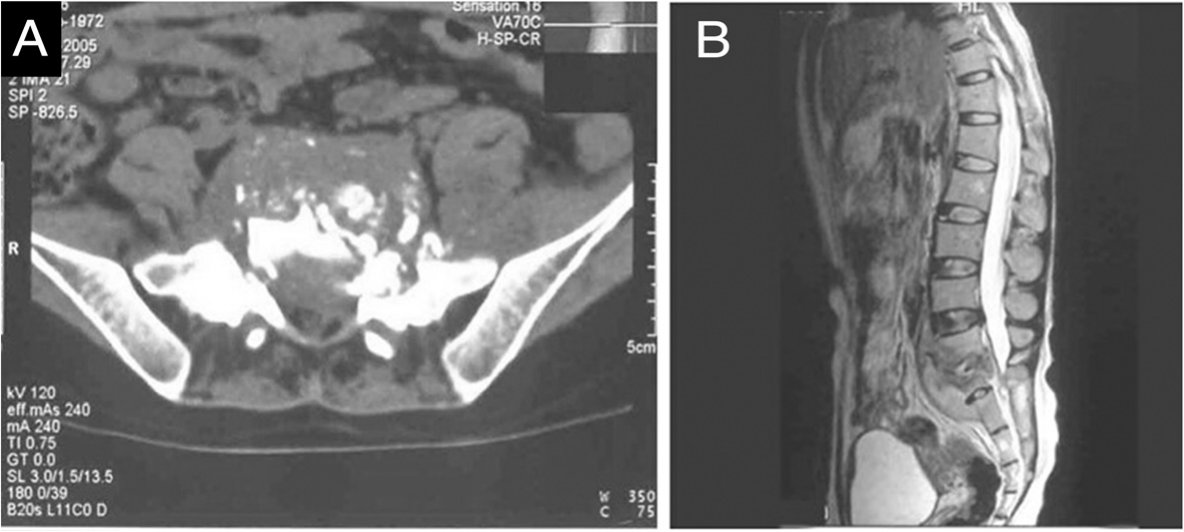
CT and MR images showing bone union of grade I. (A) CT image showing S1 bone destruction, presacral cold abscess, and sequestrum. (B) MRI showing L5–S1 vertebral signal change, narrowed intervertebral space, and presacral cold abscess.
